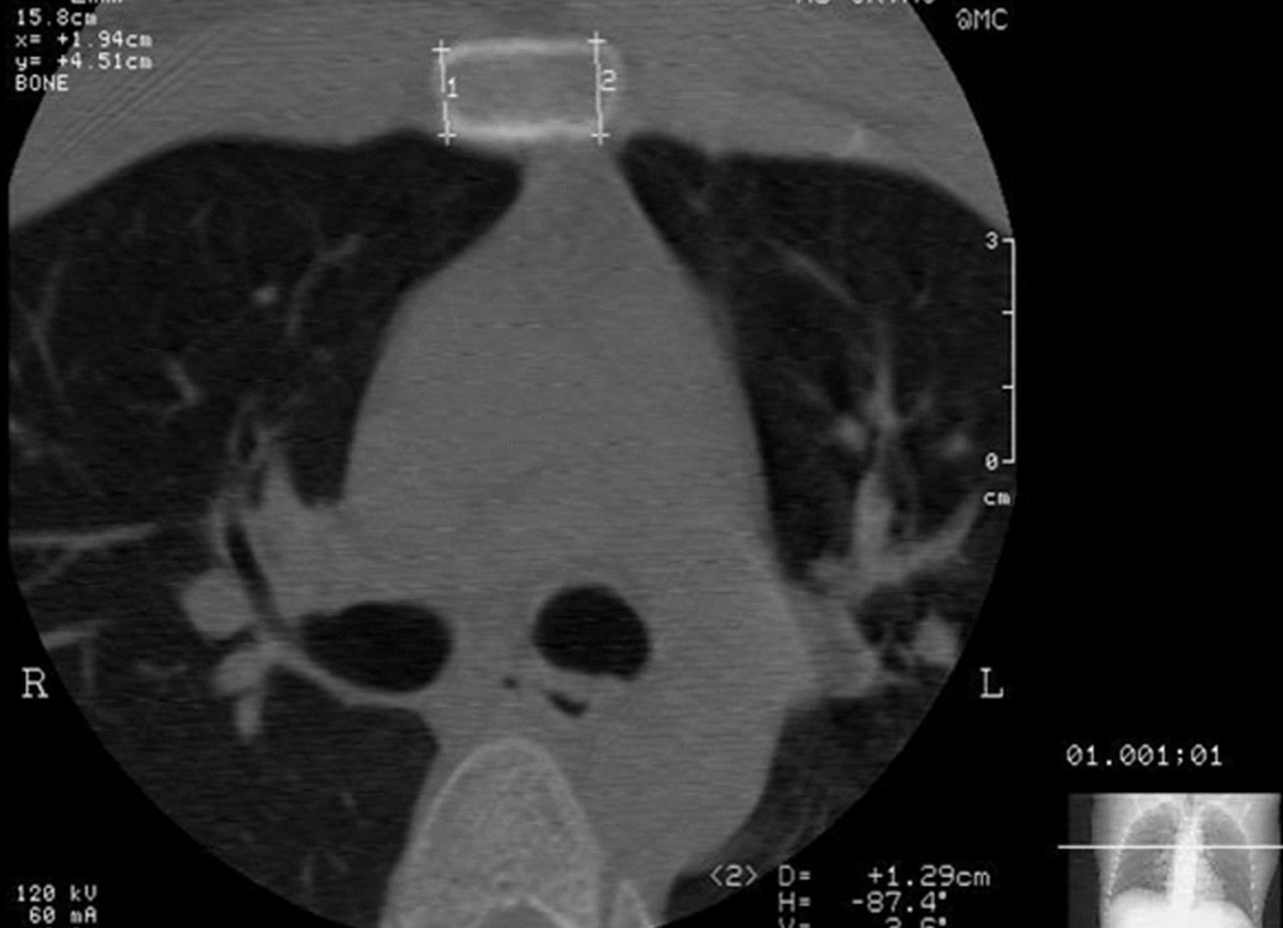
CT scan showing the measurement of thickness of manubriosternal joint.
